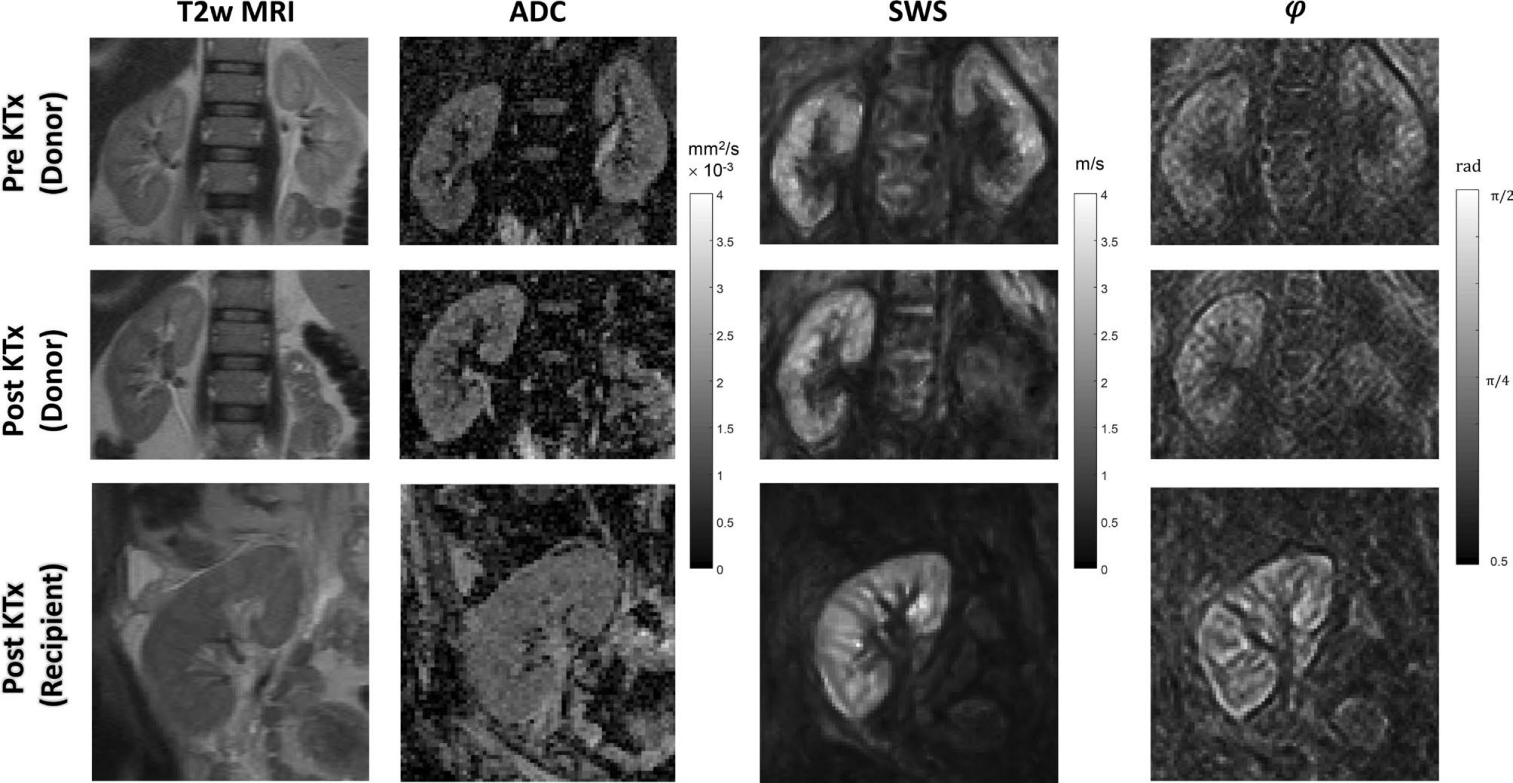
Representative images obtained in a 50‐year‐old male kidney donor and a 46‐year‐old female recipient. Shown are T2‐weighted MRI (T2w MRI), diffusion‐weighted MRI providing the apparent diffusion coefficient (ADC), and MRE providing shear wave speed (SWS) and loss angle (φ) as surrogate markers of tissue stiffness and tissue fluidity in a coronal plane.
